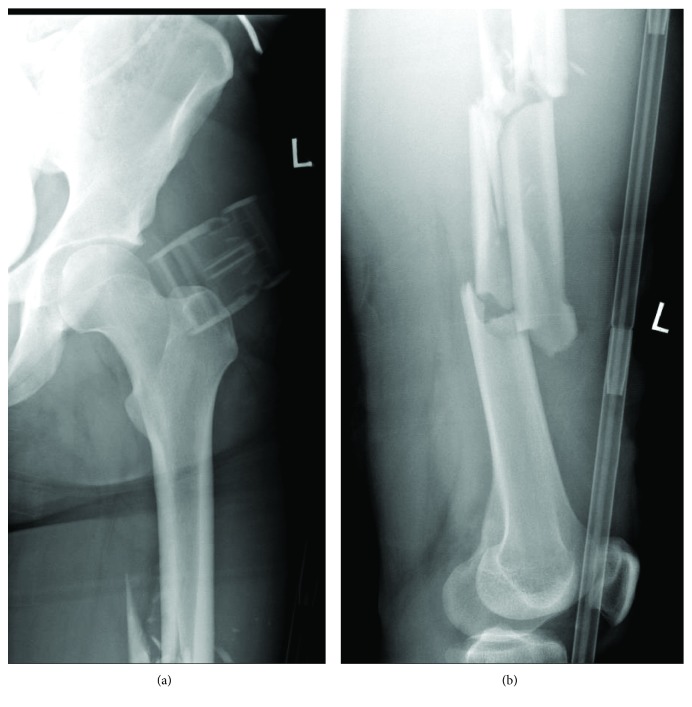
Standard anteroposterior radiograph of the left hip and femur (a); standard lateral radiograph of the left femur and knee (b).
